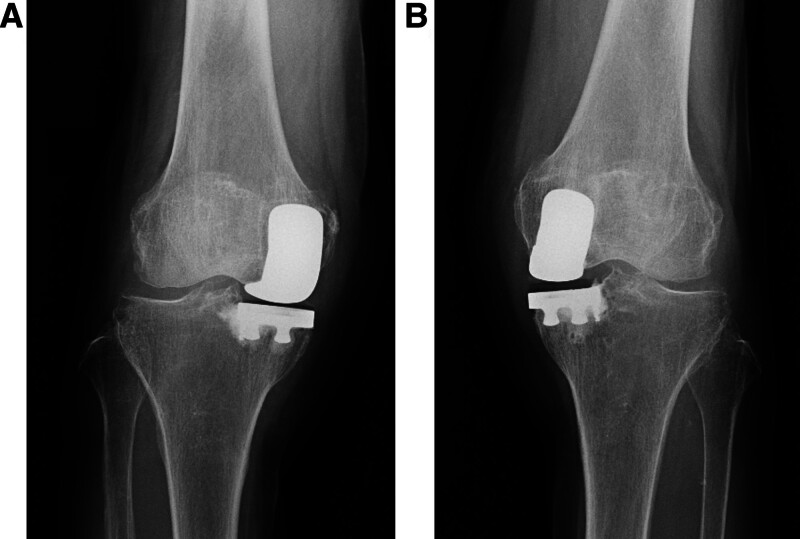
(A) The postoperative X-ray images of the right uni-compartmental knee arthroplasty. (B) The postoperative X-ray images of the left uni-compartmental knee arthroplasty.
